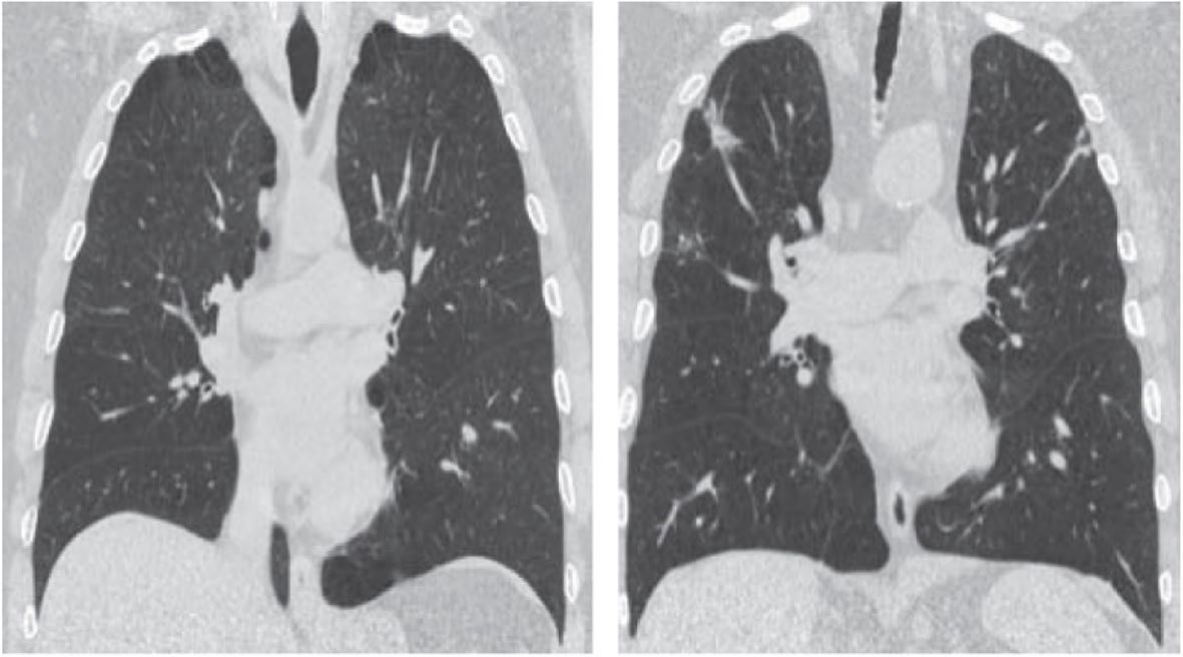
Baseline and 3^rd^ month post BLVR (CT) changes. Coronal CT imaging in a patient in each upper lobe. Scarring effect were observed
